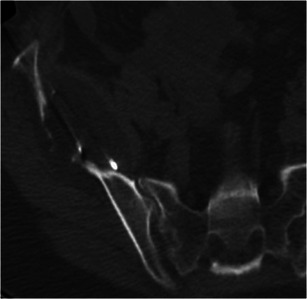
Melanoma metastasis in the right iliac bone: Cryoablation was performed with five cryoprobes inserted; notice how the iceball is visualised on the CT scan as a hypodense structure
